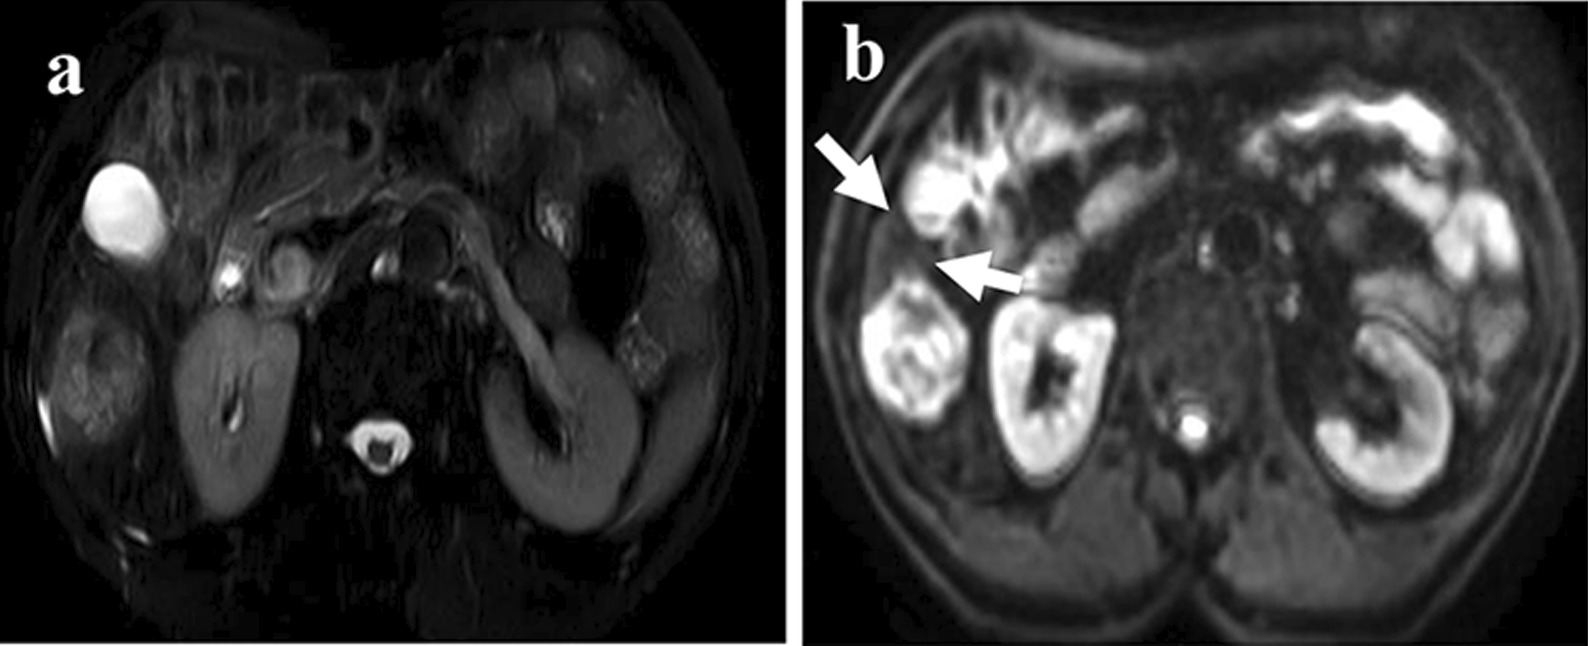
A 69-year-old woman with sHCC with MVI. a T2WI shows a high signal intensity hepatic mass with round shape and smooth margins. b DWI shows mismatch region compared to T2WI (thin arrow)
